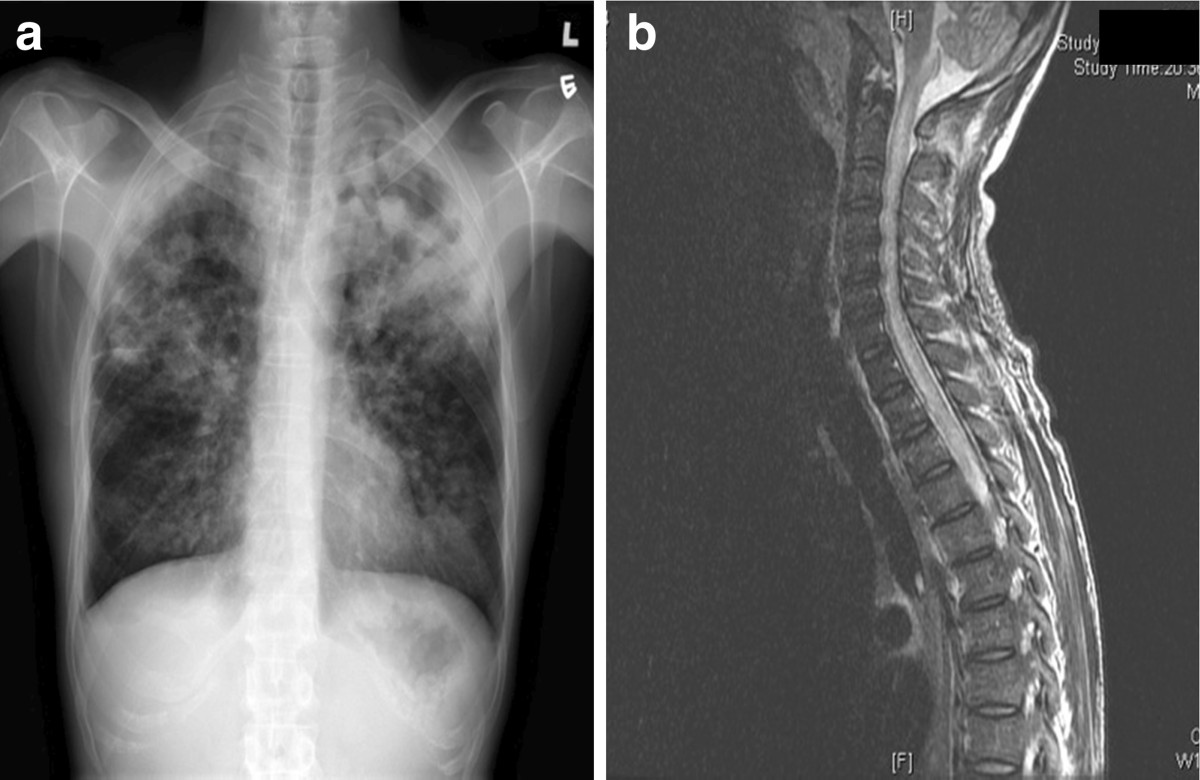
Radiological imaging of lung and spinal cord. a: Chest X-ray (PA Erect film) on initial presentation showing bilateral lung field patchy consolidation with left upper zone predominance. b: Sagittal section of MRI spine showing diffuse T2-weighted hyperintensity involving C1-T4 levels of the spinal cord suggestive of longitudinally extensive transverse myelitis (LETM).
